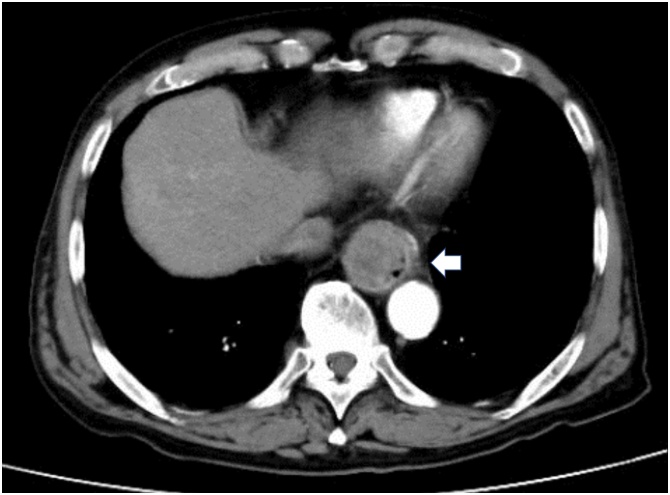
Contrast-enhanced computed tomography (CT) scans. The scan shows a 6-cm-long mass lesion with contrast enhancement in the lower thoracic esophagus (arrow).
